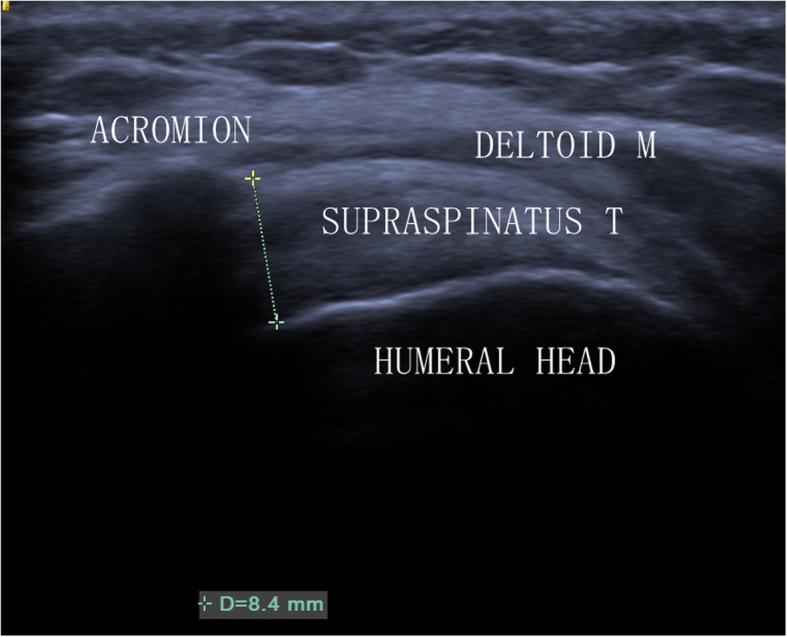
Sonogram of AHD in a normal left shoulder. AHD = 8.4 mm. T = tendon; M = muscle
